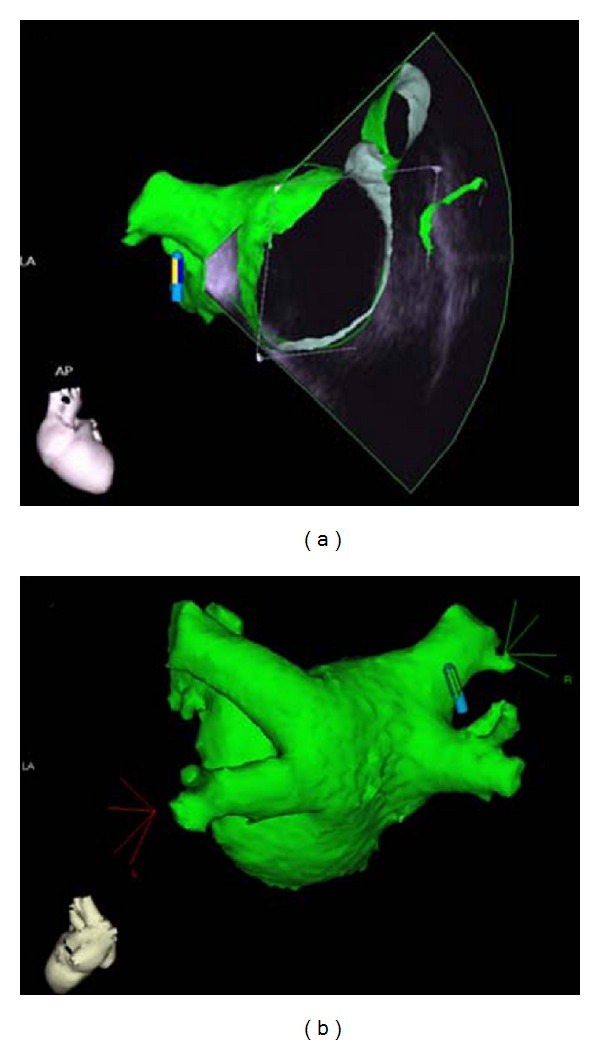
(a) ICE fan with overlying CT reconstruction of the LA in the anterior-posterior projection and (b) a completed reconstruction of the LA in posterior-anterior projection both using CARTOSound and the integrated software algorithm CARTOMerge.
